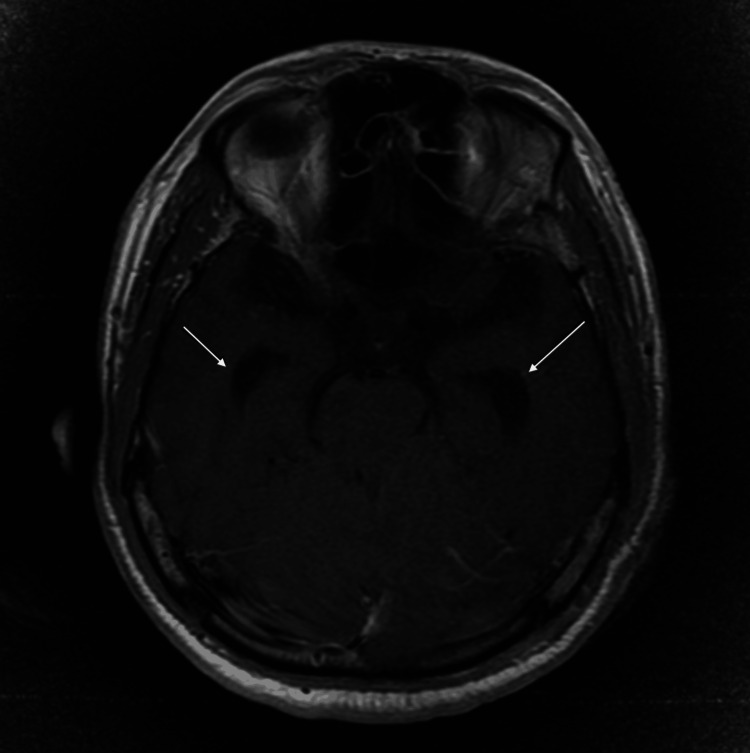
T1-weighted MRI demonstrating hydrocephalus (white arrows)
